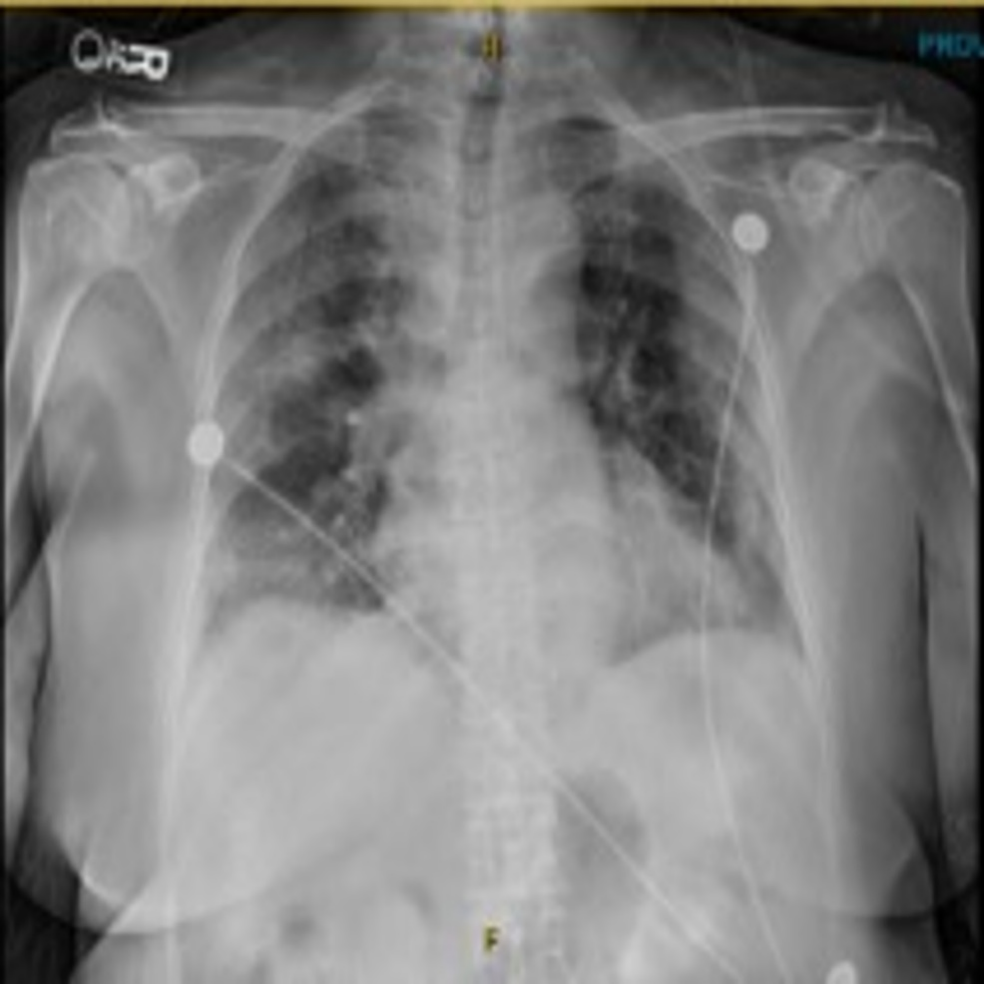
Chest X-ray on admission. Chest X-ray on admission showing bilateral ground-glass opacities consistent with COVID-19 infection.
